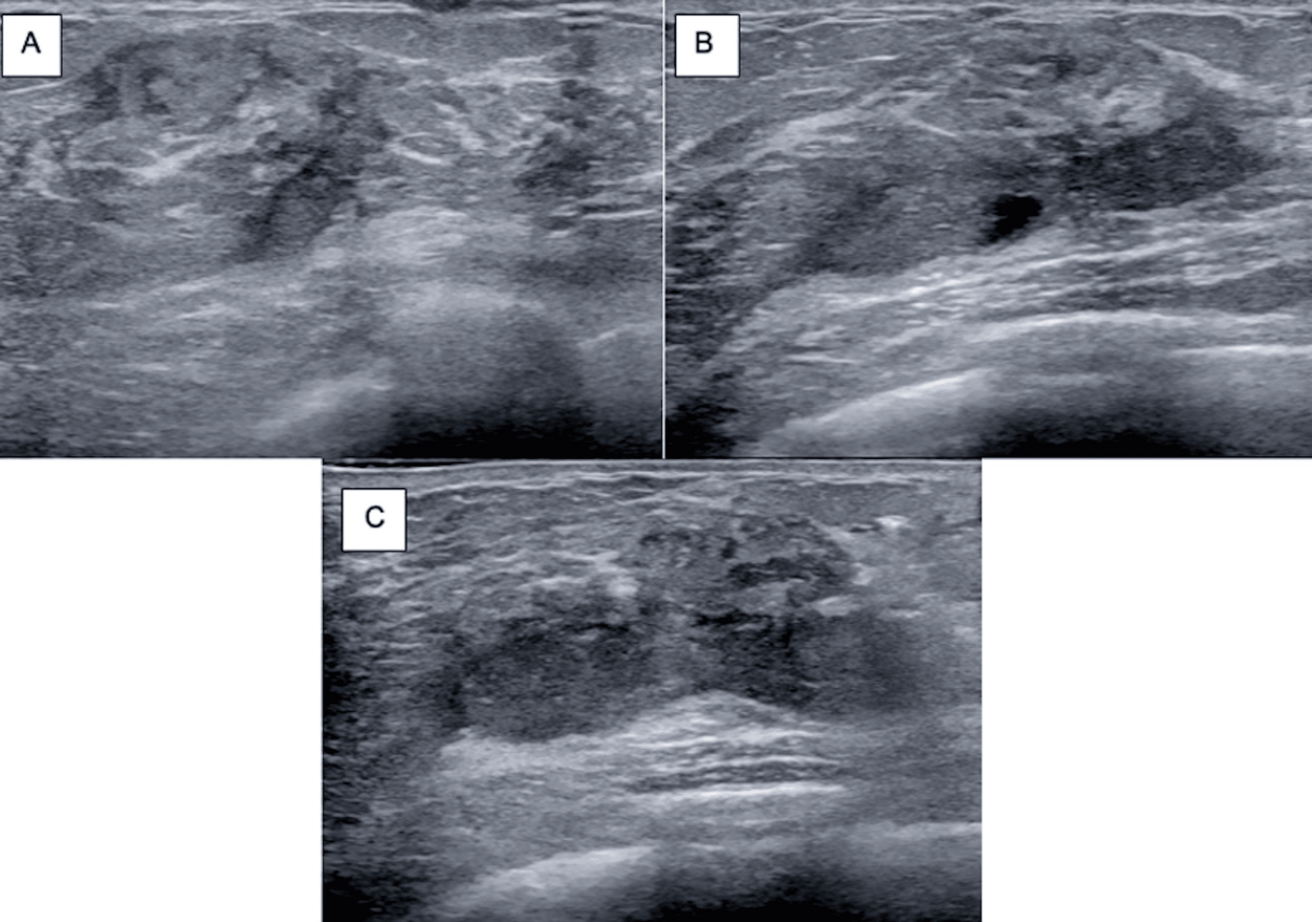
Ultrasound at the two-month follow-up: (A) antiradial, (B) radial, and (C) oblique view. Targeted ultrasound of the left breast performed at the time of ultrasound-guided biopsy, approximately two months after initiation of treatment at the initial visit, demonstrated the previously identified lesion at the biopsy site.
